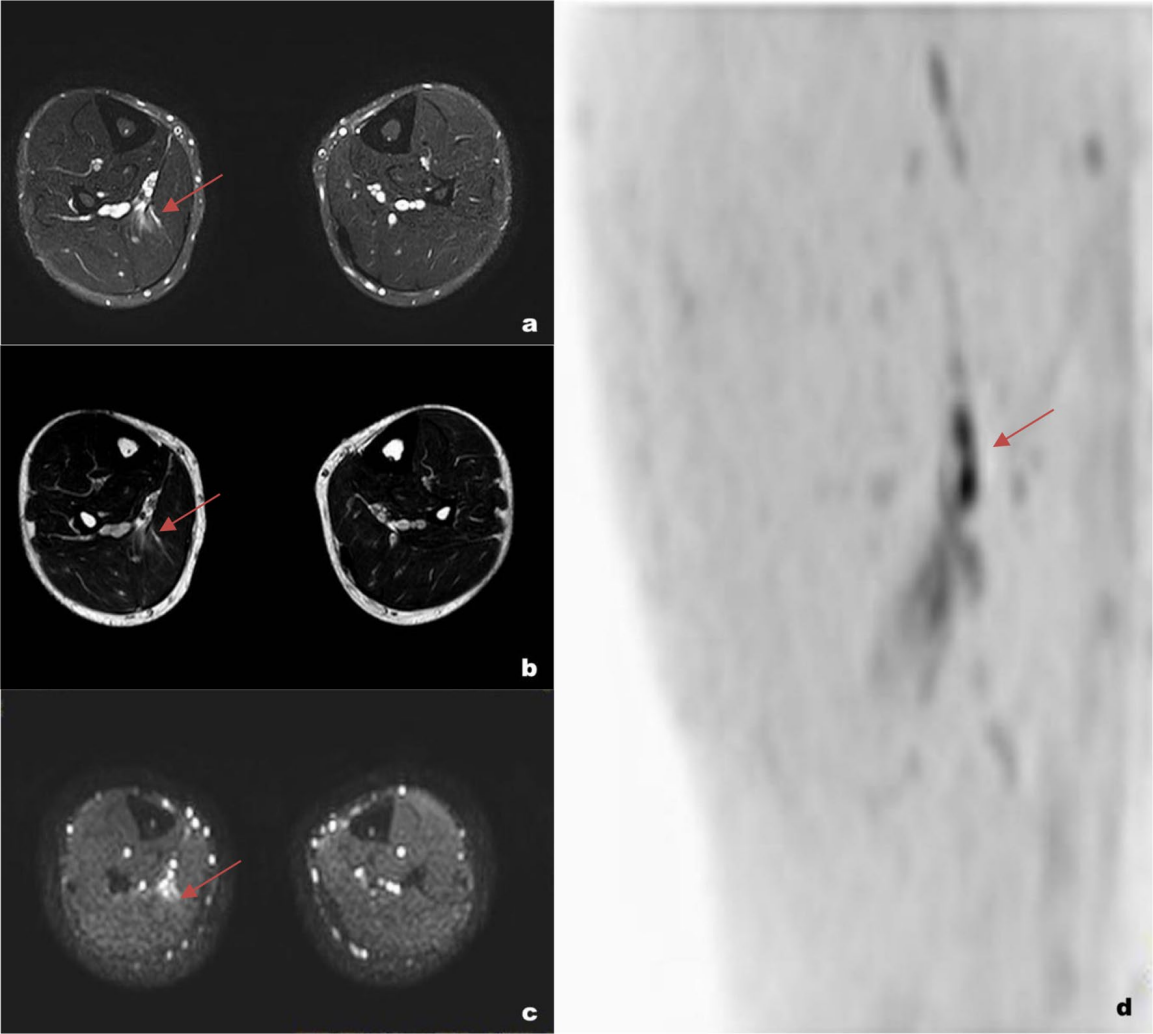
a 26-year-old athlete with a myotendinous lesion in the middle third of the right soleus (red arrow). The Axial TSE dual proton density-weighted SPAIR (a) and axial TSE dual proton density-weighted images without fat suppression (b) show both intramuscular edema and muscle fibers interruption at the myotendinous junction. The DWI images (c) allow a clearer representation of the actual muscular injury (hyperintense spot). The DWI volume reconstruction (d) allows the precise evaluation of lesion craniocaudal extension. The lesion was classified as 3a using the Mueller-Wohlfahrt classification. The value on the ADC map was 1.6·10^–3^ mm^2^/s
